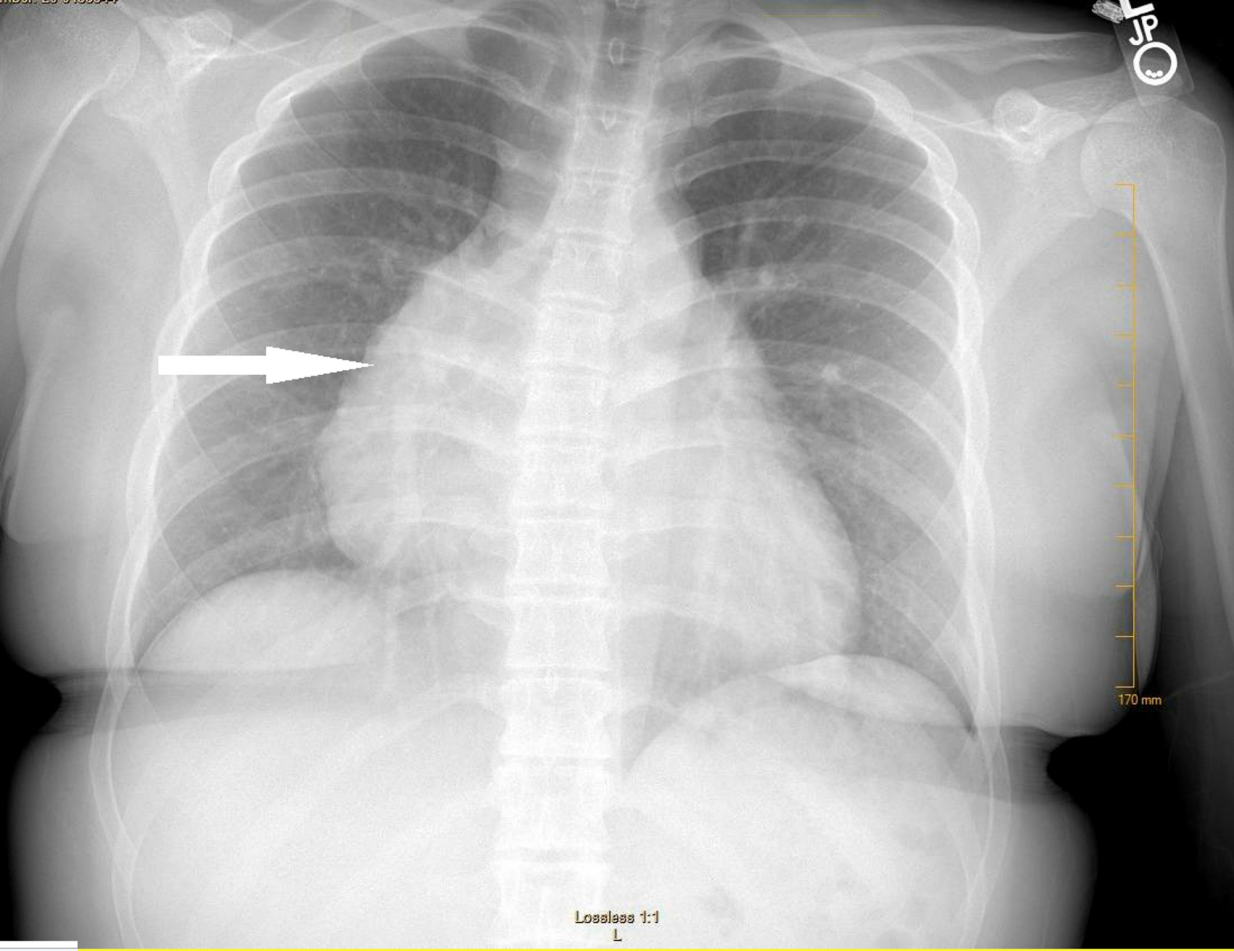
Portable anteroposterior chest x-ray demonstrating cardiomegaly (white arrow) without pleural effusion, pneumothorax, or focal consolidation
